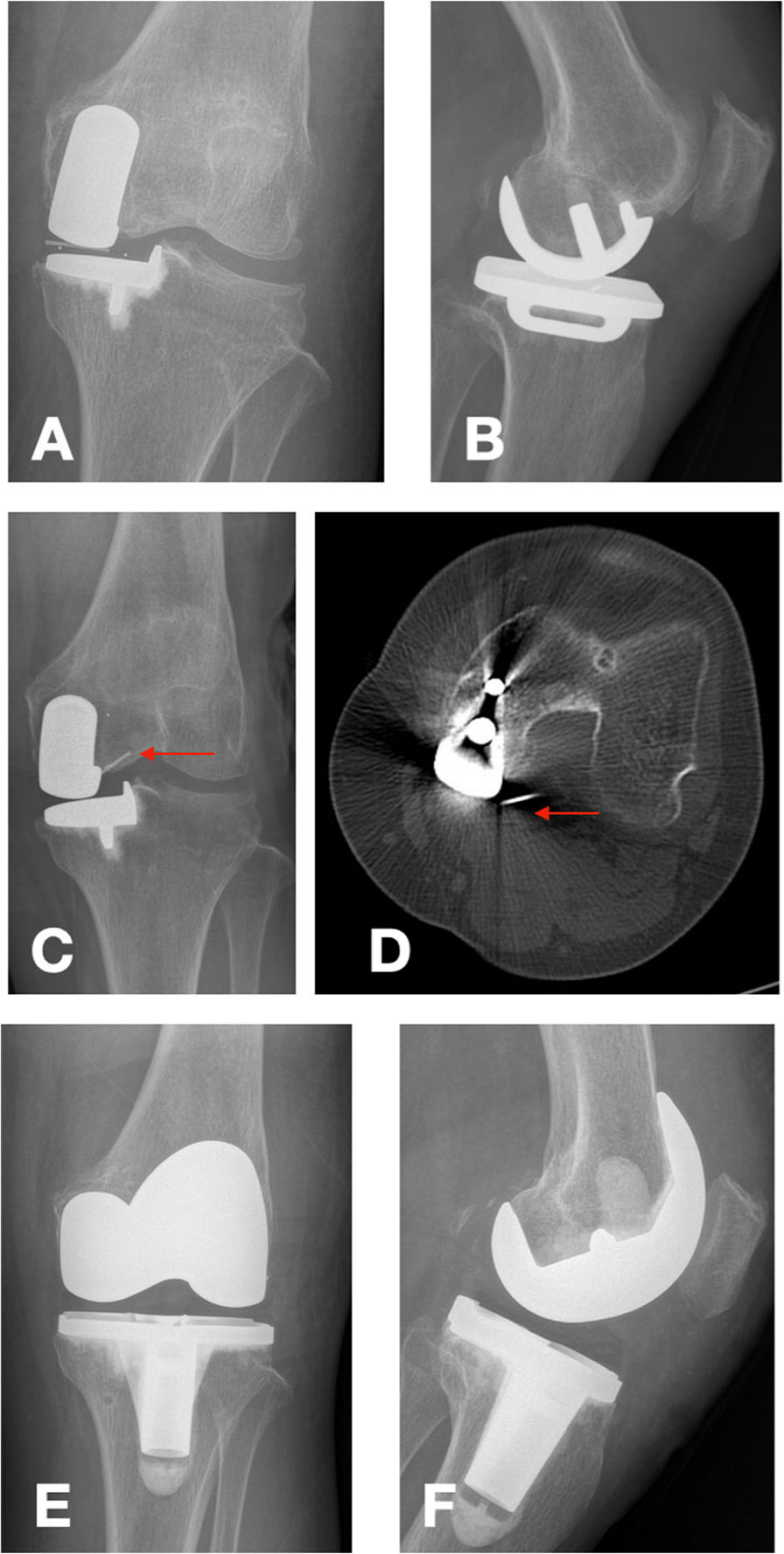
shows a 64-year-old woman suffered moderate pain 4 months after surgery, and the patients received X-ray and CT scan for examination. From the data of imaging we found bearing dislocation. Later the patient performed TKA and got satisfactory clinical outcome after TKA. a and b are the X-rays of the patient 2 weeks after surgery. c is the X-ray of the patient 4 months after surgery. d is the CT scan of the patient 4 months after surgery. e and f are the X-ray of the patient 1 year after TKA surgery
